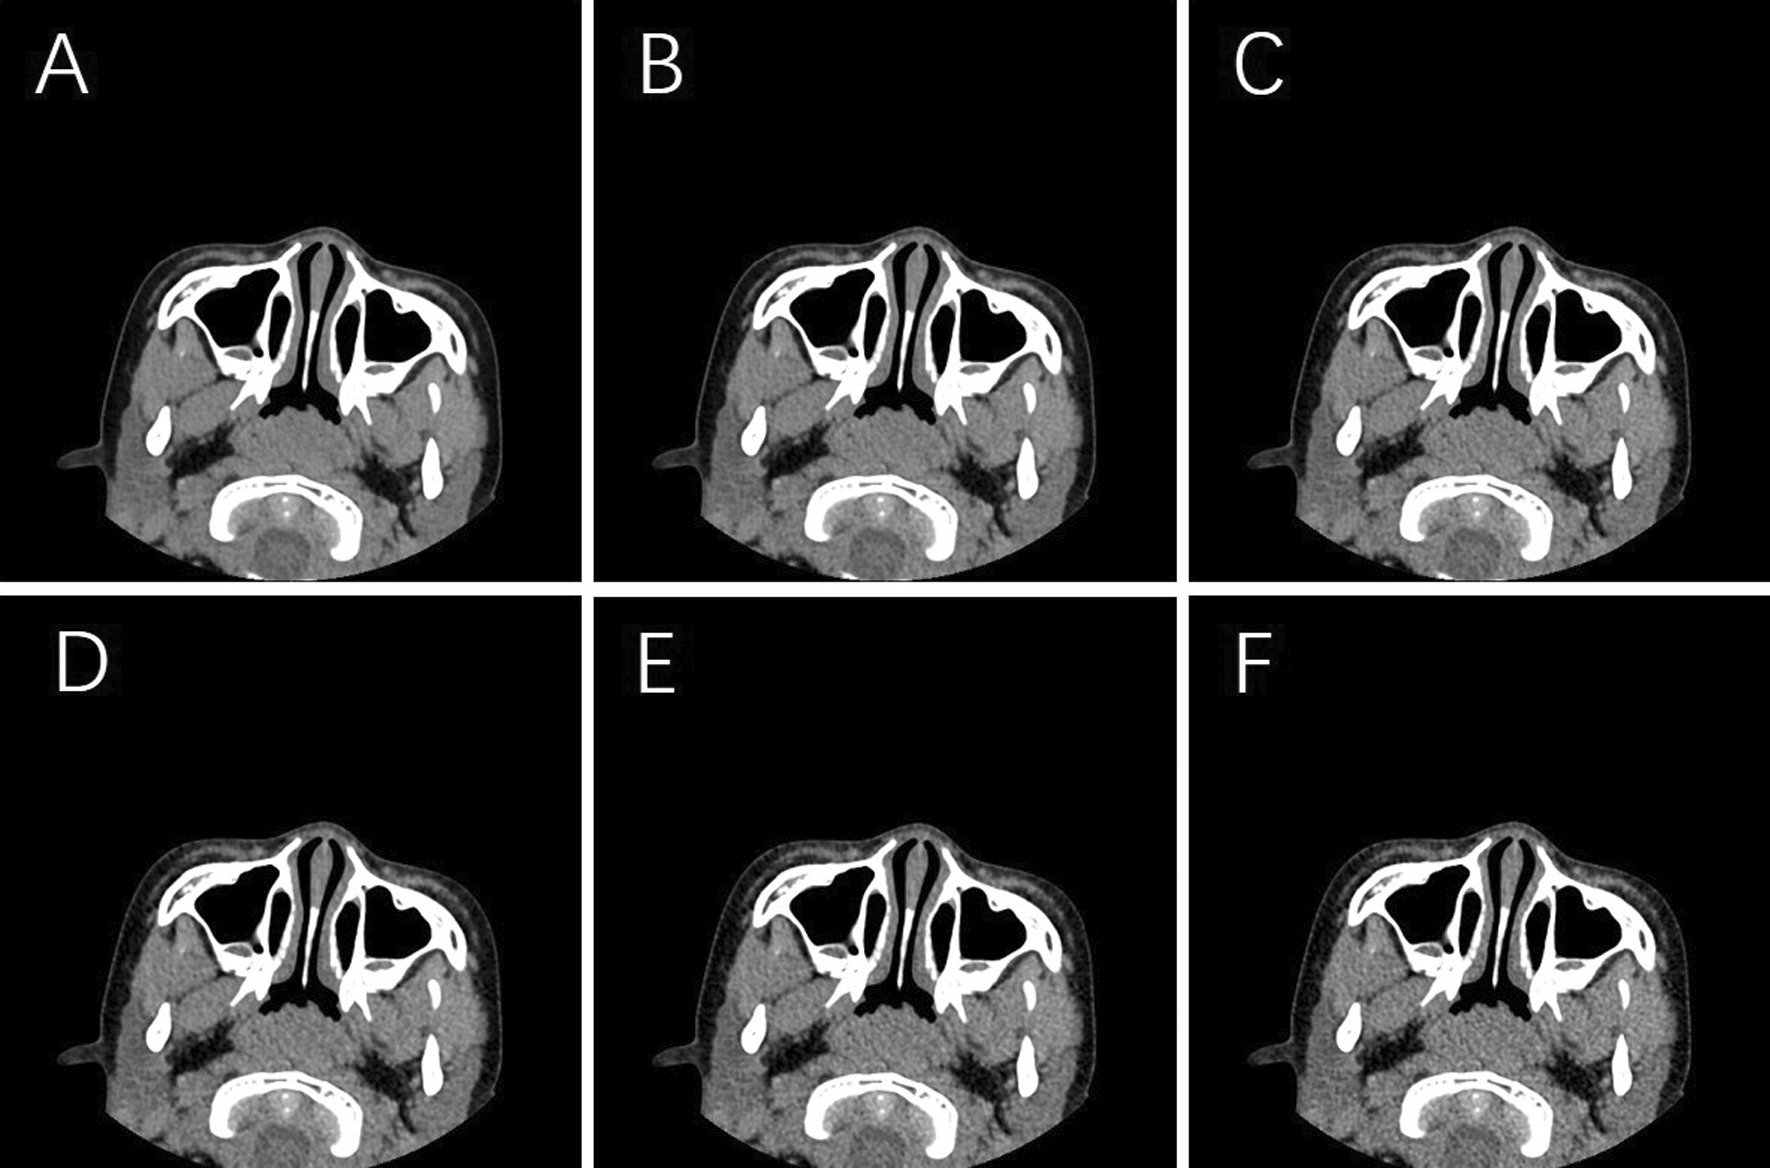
Axial CT soft tissue window images from different reconstruction algorithms. A DLIR-high, B DLIR-medium, C DLIR-low, D AsirV-50%, E AsirV-30%, F FBP. The subjective image quality, mainly evaluated based on image sharpness and graininess, was ranked, in descending order, as DLIR-high, DLIR-medium, DLIR-low, 50% post-ASiR-V, 30% post-ASiR-V, and FBP
